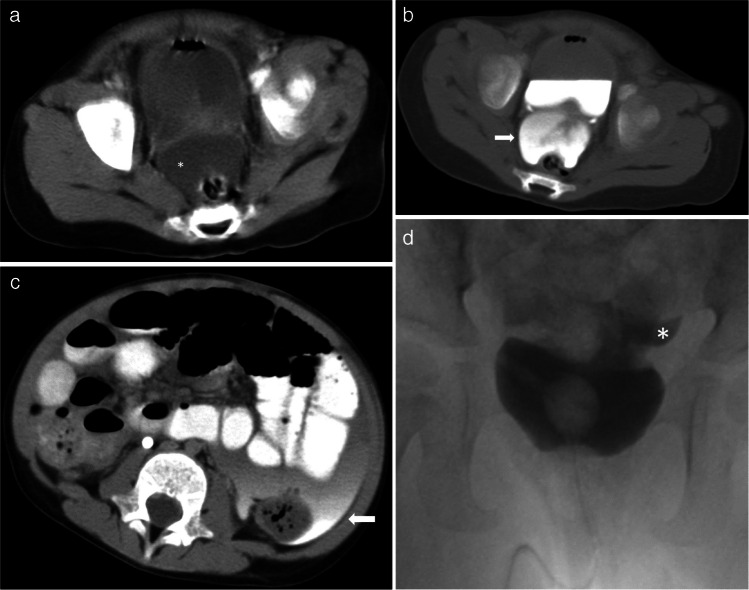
A 2-year-old female presented with abdominal bruising, abdominal pain, and acute renal failure without a history of trauma. Findings elicited concern for physical abuse. a Axial image at the level of the bladder in routine portal venous phase shows low-density fluid in the cul-de-sac (*white asterisk*). Air in the bladder from a recent Foley catheter placement is also visible (*unmarked*). b Axial CT image at the level of the bladder in excretory phase demonstrates contrast opacifying the cul-de-sac fluid consistent with urine leak (*white arrow*). c Axial CT image of the same study in excretory phase at a more cephalad location from the bladder shows contrast extravasation into peritoneum (*white arrow*). d Cystogram performed on the same patient through a Foley catheter shows contrast leakage from the bladder revealing an intraperitoneal bladder rupture (*white asterisk*)
